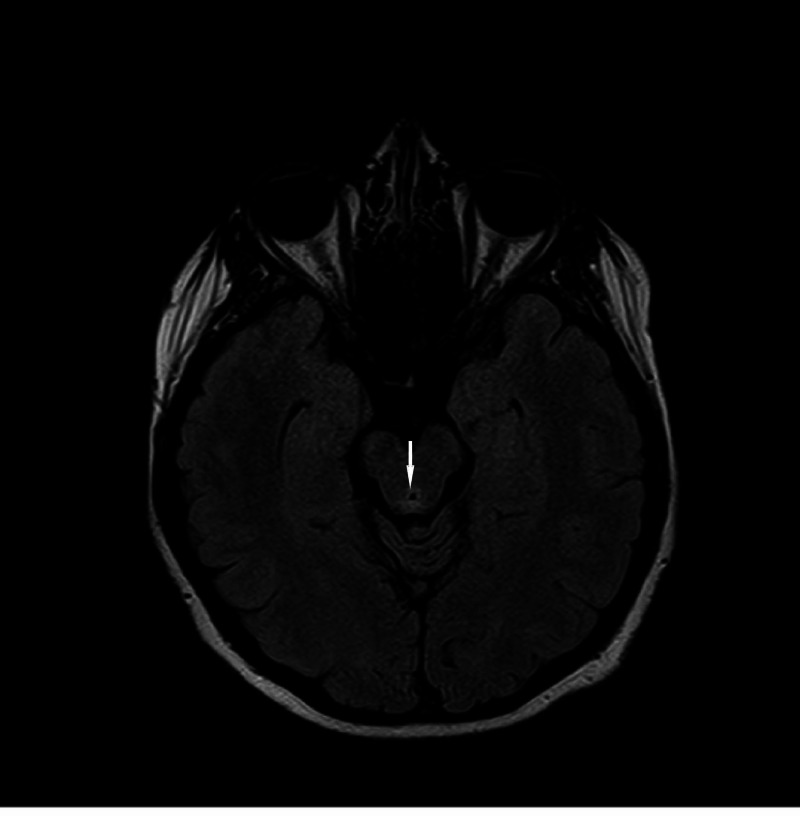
MRI axial T2 fluid-attenuated inversion recovery (FLAIR) brain image showing hyperintensity in periaqueductal gray area.
